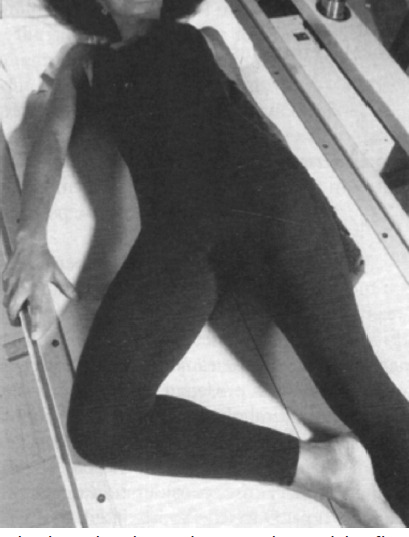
The lateral radiograph were obtained by flexing the index knee, with both the knee and ankle being horizontal to the floor and touching the table top.
